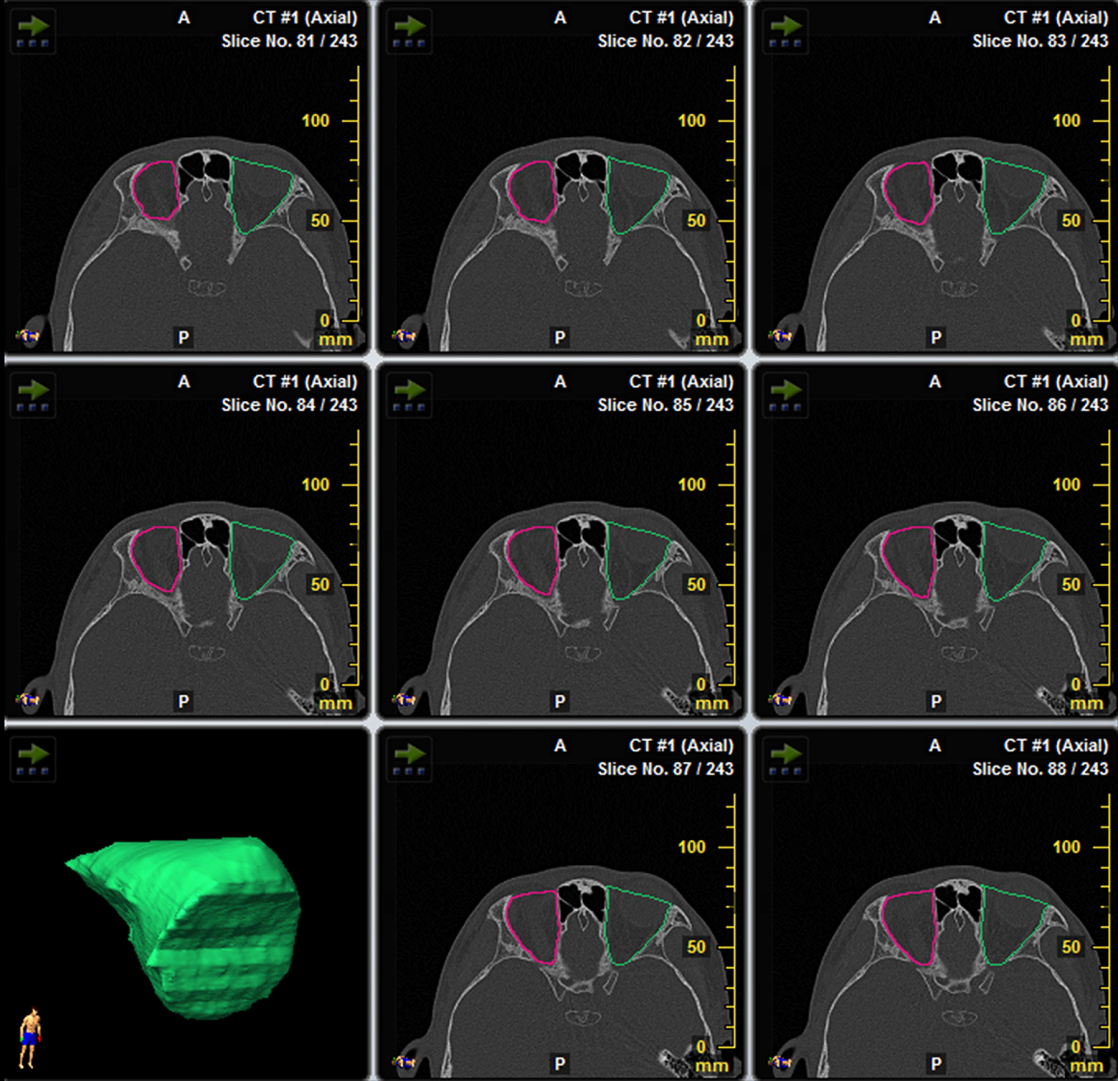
Measurement of orbital volume by iPlan Cranial software. The bony orbit was outlined in each slice of axial CT scans. The software generated 3D reconstruction of the orbit and calculated the orbital volume.
